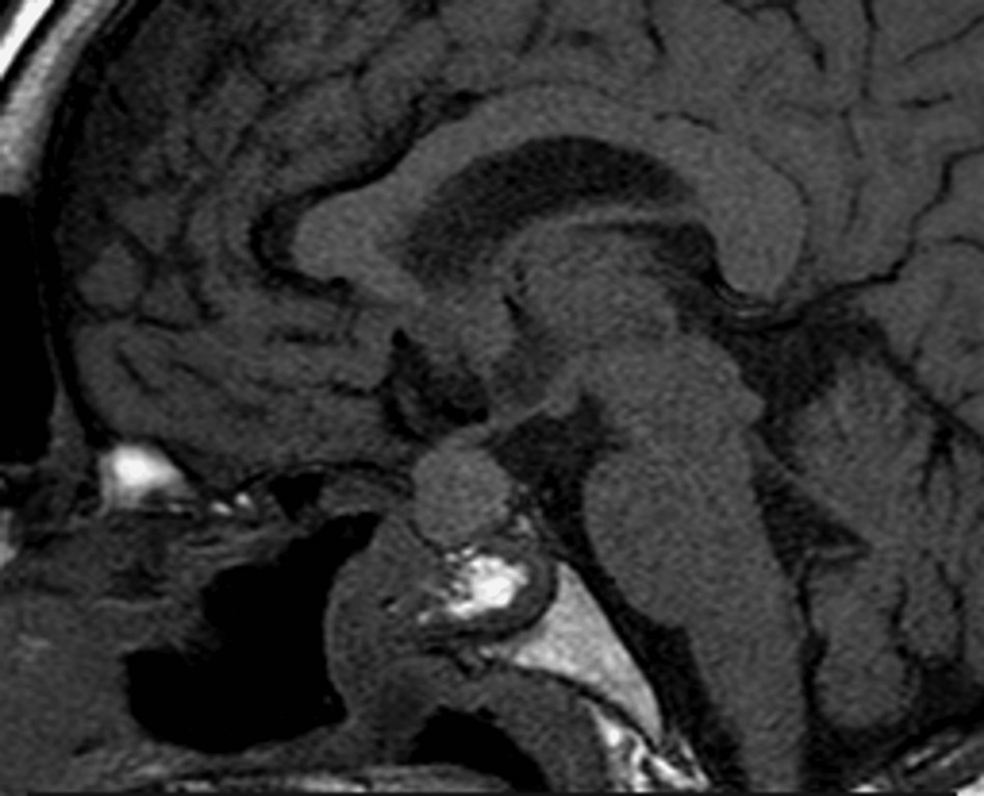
Saggital T1-weighted MRI image showing an isointense lesion in the sellar and suprasellar region
